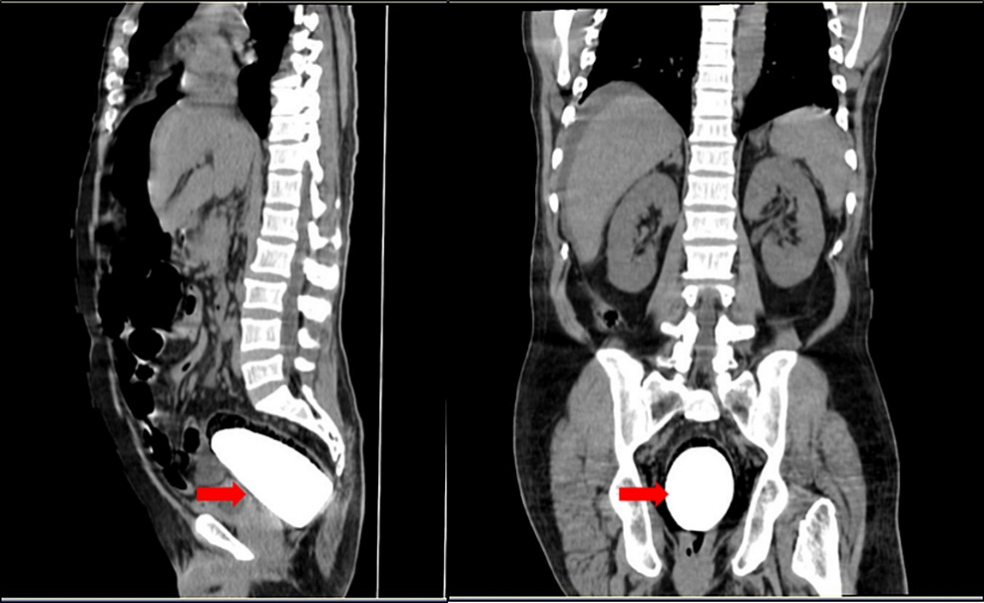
CT abdomen - sagittal and coronal views demonstrating what looks like a "pestle" in the rectum.
